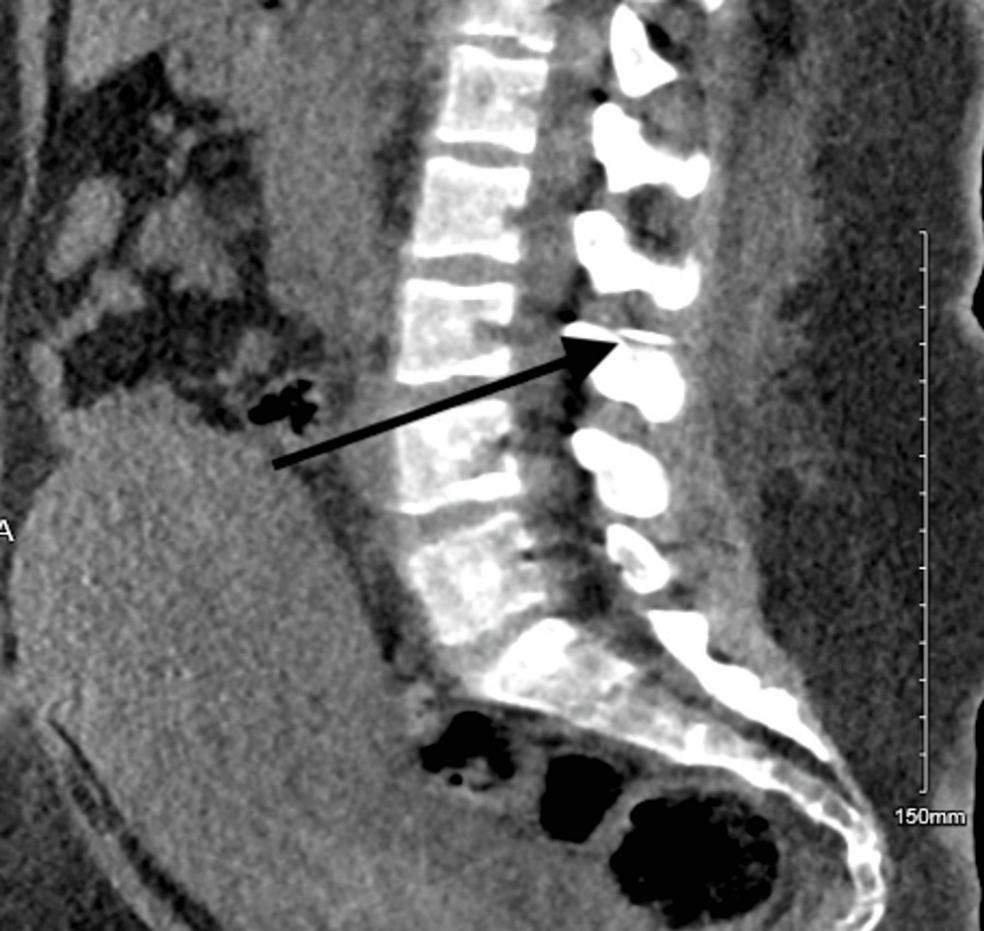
CT scan of the spine at the lumbar (at the L4 level). The CT imaging sequence indicates the epidural catheter's entrance and where it ends. In this image, you can see the catheter ending at the level of the L4 endplate.
